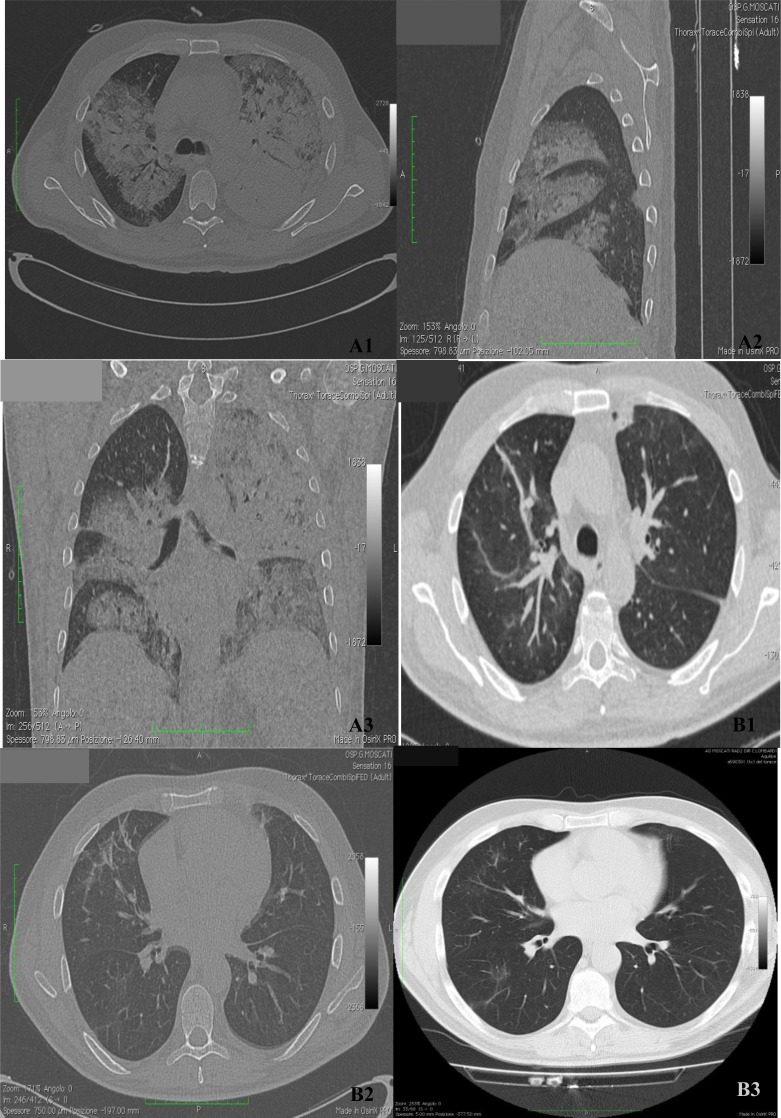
Chest CT images. At the ER, the chest Ct highlights the bilateral multiple foci (A). After 7 days there is the important regression of the radiological evidence (B).
